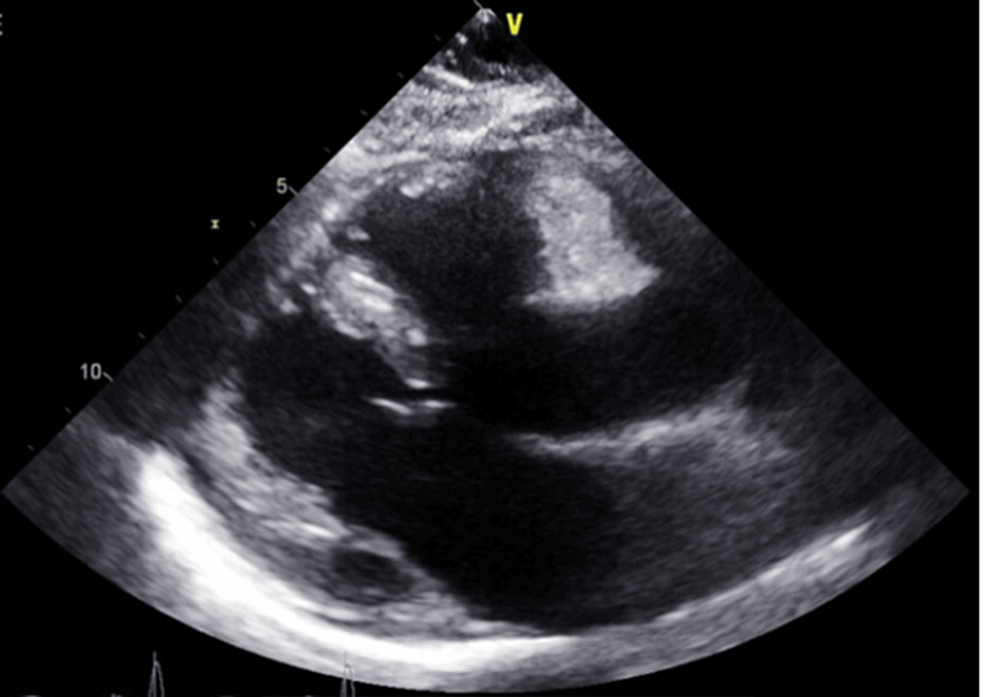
Initial transthoracic echo on admission showing no pericardial effusion
